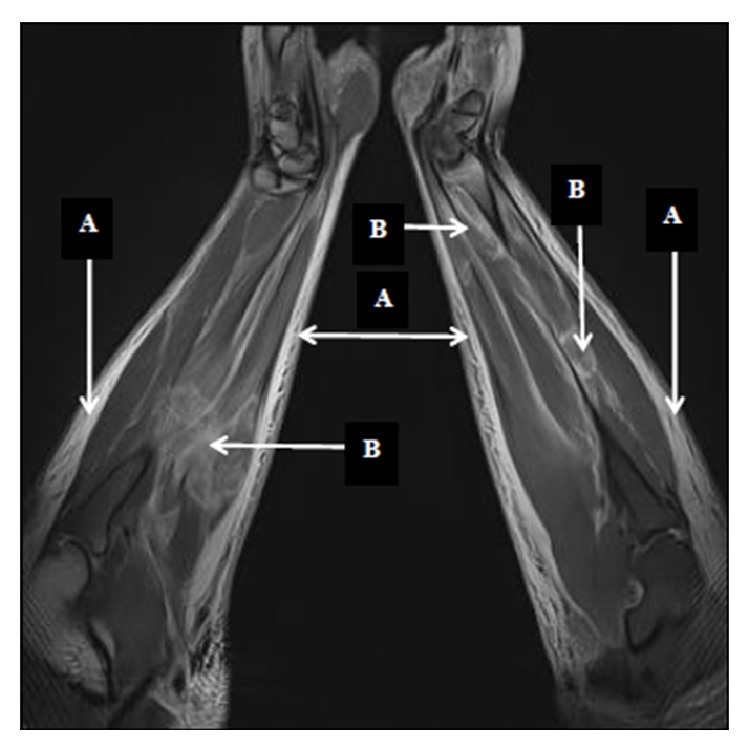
Coronal fat-suppressed proton density-weighted MR images of both forearms show diffuse hyperintensity in the subcutaneous fat tissue (A) and multifocal, confluent areas of hyperintensity in the muscles due to edema (B).
